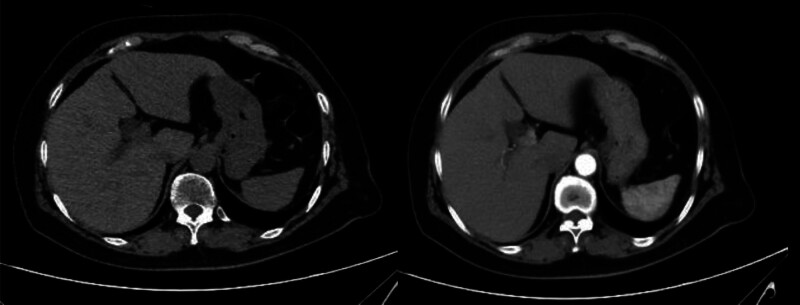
Abdominal CT scan. CT = computer tomography.
